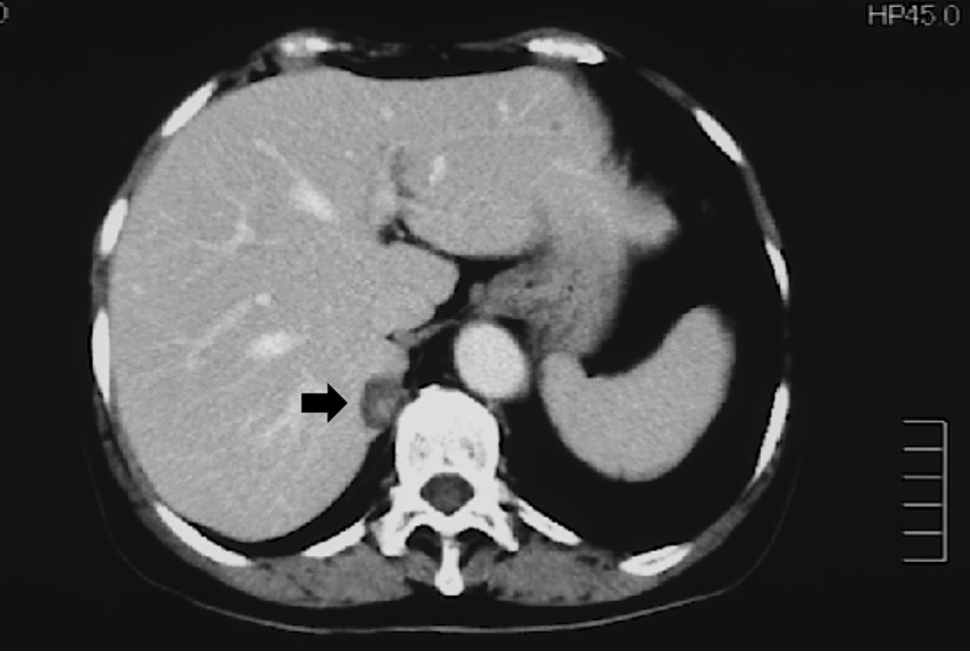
Computed tomography of the abdomen showed a right adrenal tumor of 2.0 cm in diameter (*arrow*)
